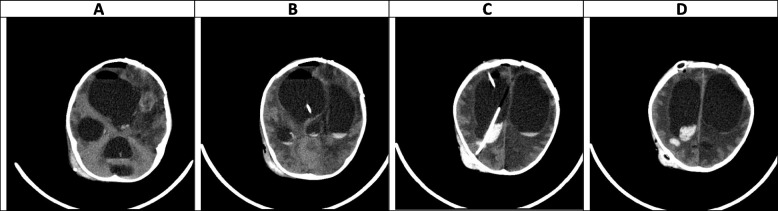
(the Brain CT SCAN without contrast, day 102). A-D Some air anterior to the right cerebral hemisphere and within the right lateral ventricle is seen, which is due to a recent operation. shadow of two EVDs is seen traversing the cerebral hemisphere and entering the right lateral ventricle. Some hemorrhage is seen along the path of the posterior EVD which is due to a recent operation. Also, some hemorrhage is seen within the ventricular system which can be due to recent EVD insertion and partly due to the extension of previous hemorrhagic lesions to the ventricular system. Despite the presence of mentioned EVD, sign of the severe communicating type of hydrocephalus is seen, associated with a mild shift of midline structures to the left side. Also sign of previous hypodense lesions and associated diffuse edema formation within the white matter is again seen in this study
